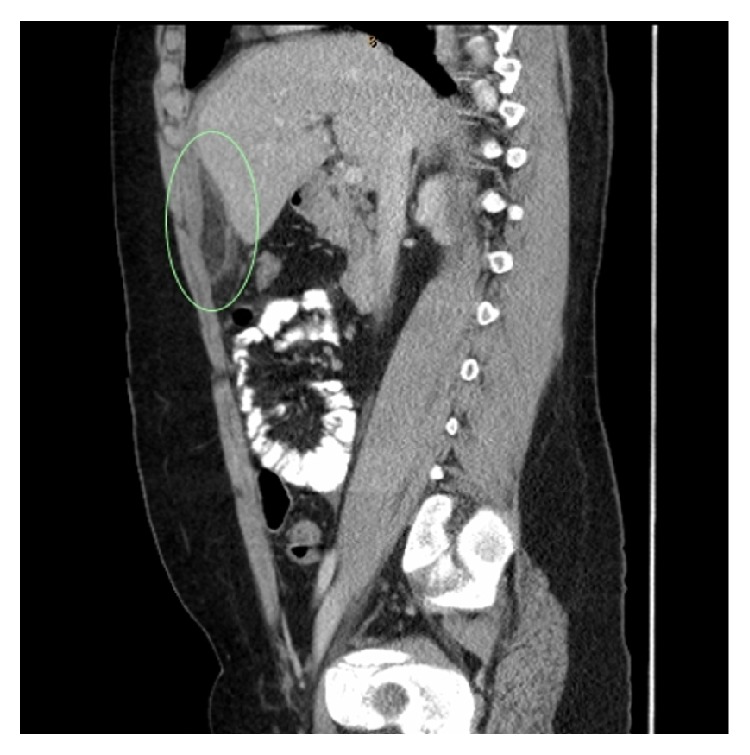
CT abdomen and pelvis-sagittal view. Torsion of lipomatous appendage of falciform ligament circled.
